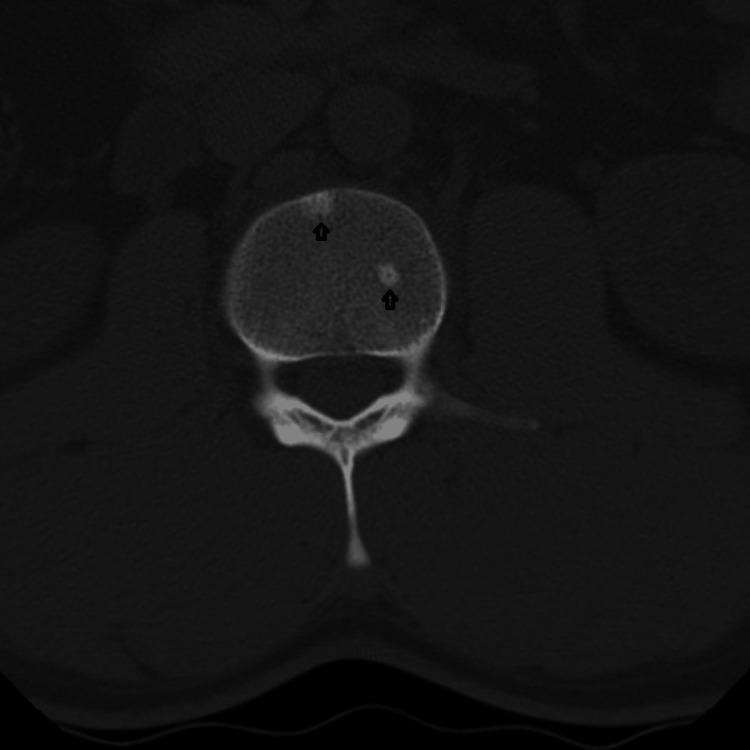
Axial section of L2 vertebra, with two osteoblastic metastases on plain CT scan
